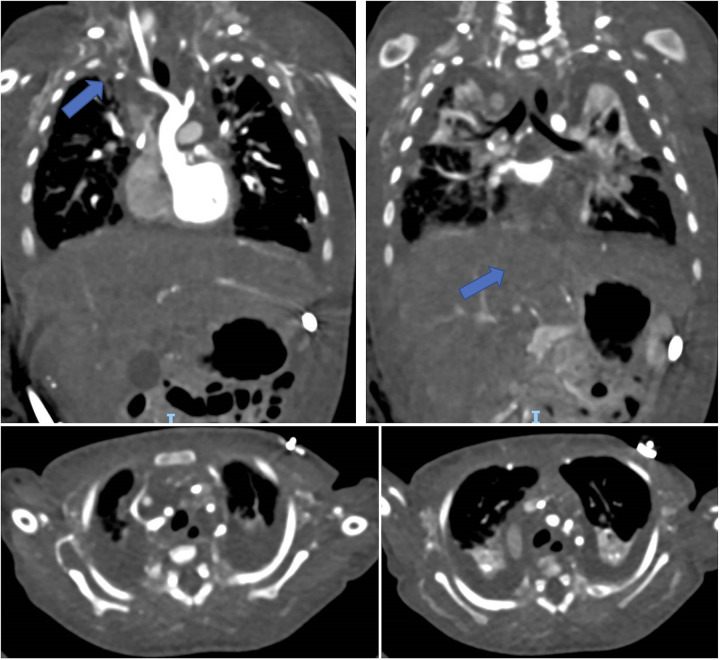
CT imaging demonstrating thrombosis of the inferior vena cava and thrombosis of the right subclavian vein.
